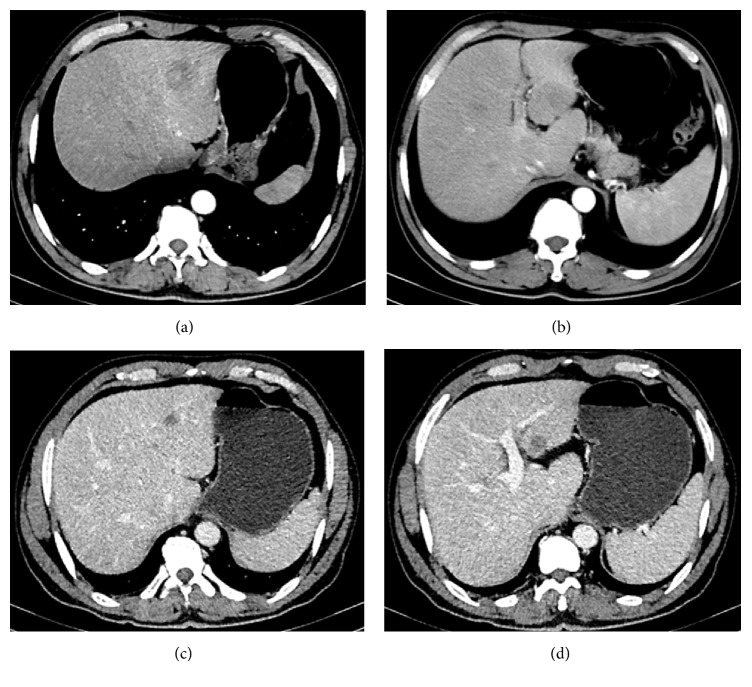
Initial abdominal computed tomography scan (a, b) and follow-up scan after three cycles of EOX chemotherapy (c, d). (a) Scan shows that the metastatic lesion in liver segment 4; (b) hypodense metastatic lesions in the left lobe of liver; (c) scan shows that the metastatic lesion in liver segment 4 has decreased in size from 2.8 cm to less than 0.9 cm; (d) scan shows that the metastatic lesion in the left lobe of liver has decreased in size from 4.3 cm to less than 1.5 cm.
